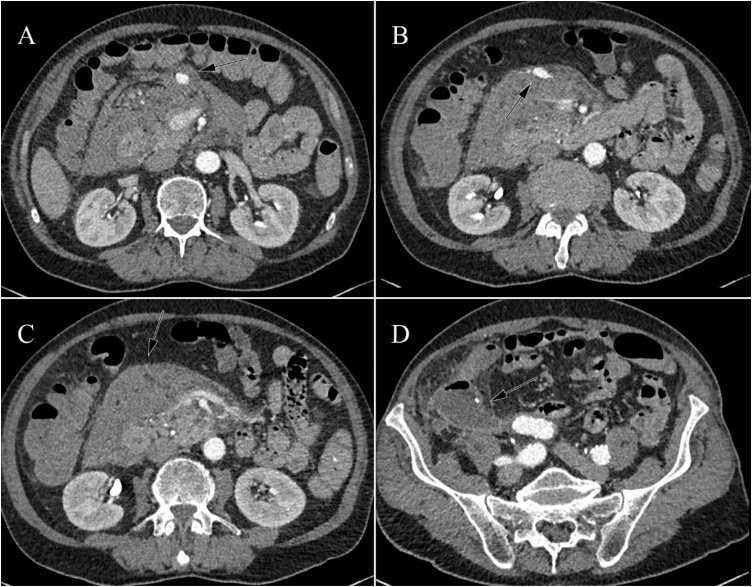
CT angiography scan showing multiple aneurysmatic dilatations (A and B), a panniculitis like infiltrating mass (C) and a new abscess in the right lower abdomen (D).
